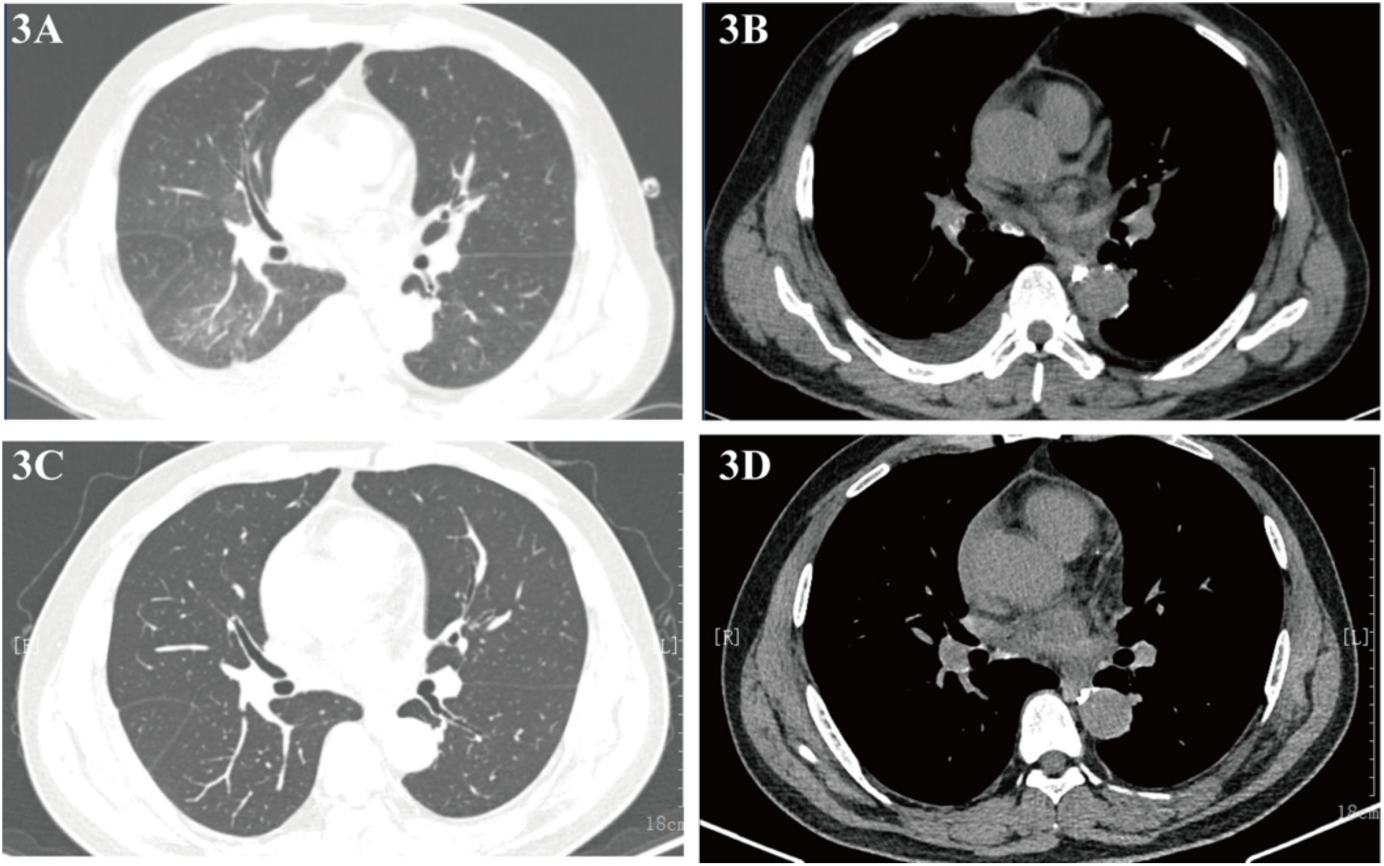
(A,B) Chest CT (September 4, 2023) showing reduced infiltrates and effusion; (C,D) follow-up CT (October 25, 2023) confirming resolution.
